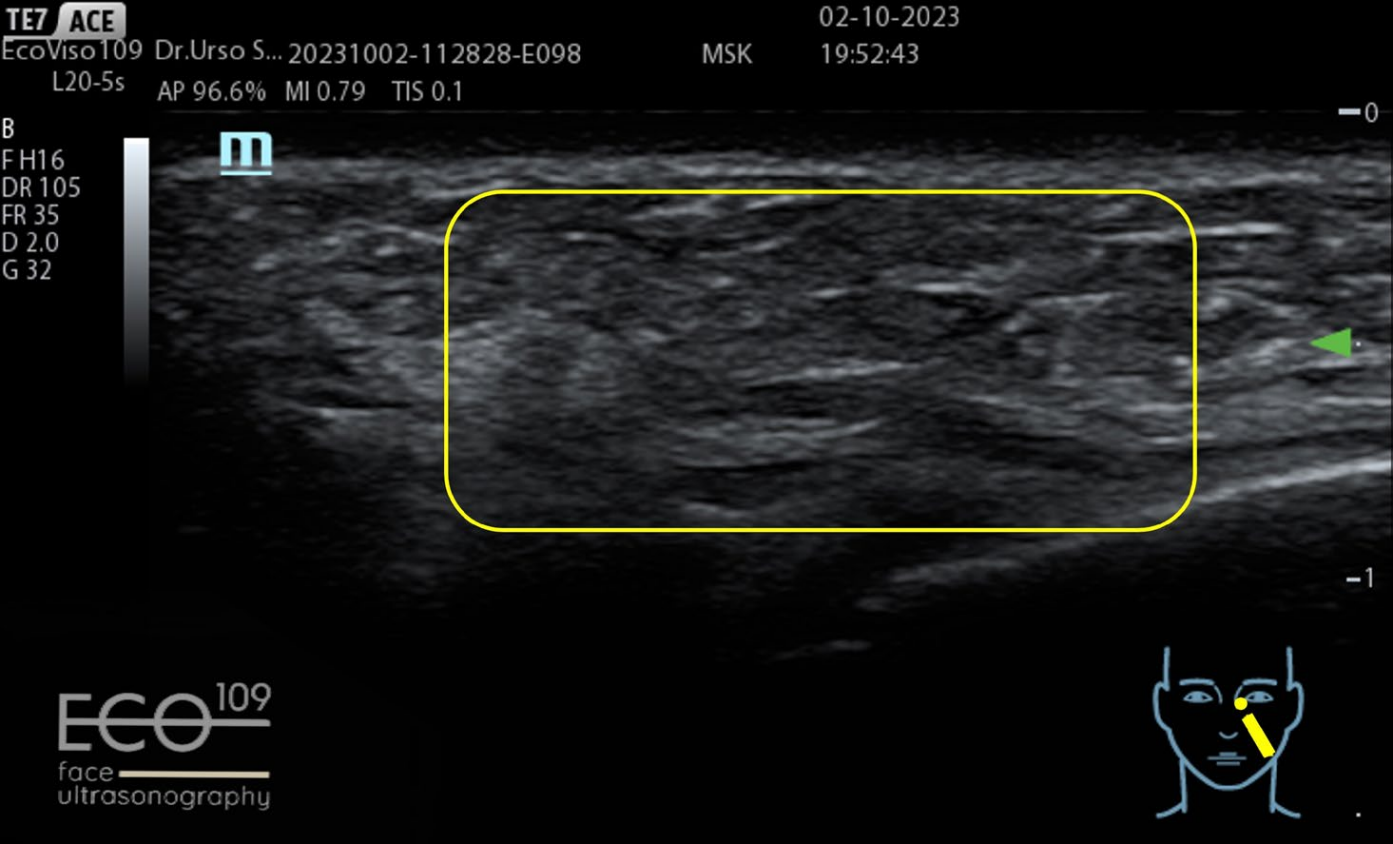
Before: Normal dermal and subcutaneous ultrasound stratification is observed.
